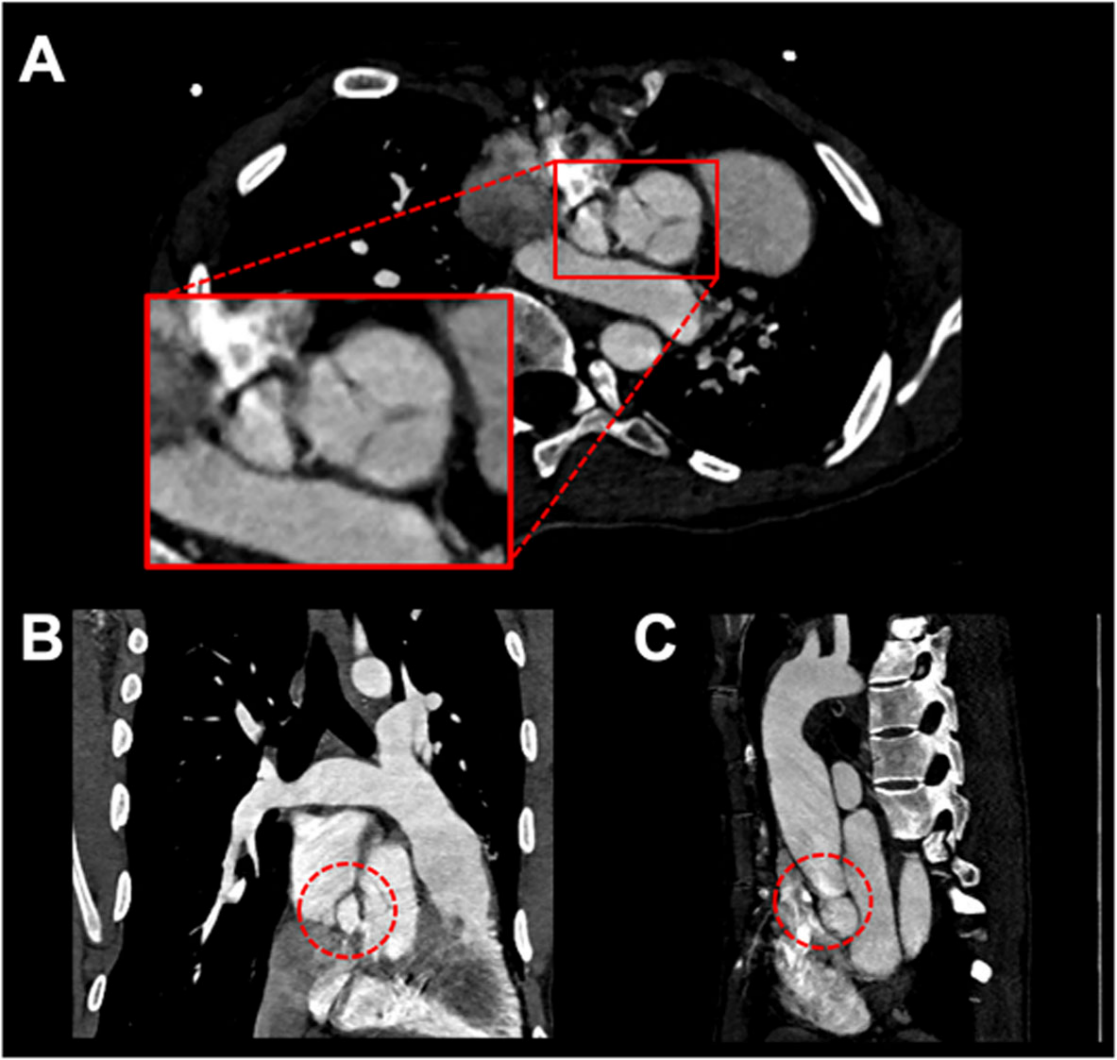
Cardiac Computed tomography (a: axial, b: coronal and c: sagittal reformats) demonstrates an enlarging 20 × 17 × 12 mm pseudoaneurysm arising from the aortic annulus surgical anastomosis caudal to the non-coronary cusp with neck measuring 3 mm post Bentall procedure (red circle)
